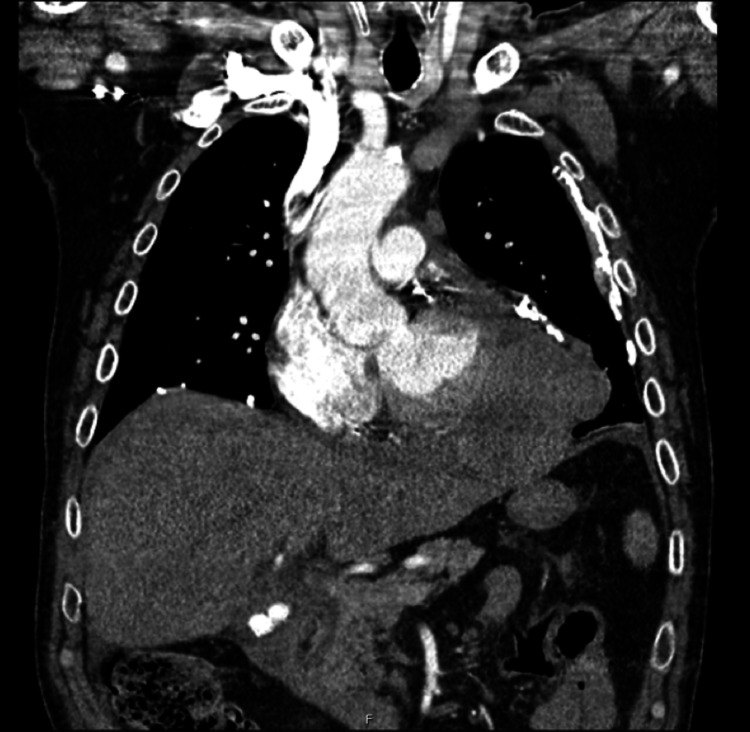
Coronal CT image showing pericardial mass with probable ventricular wall invasion
